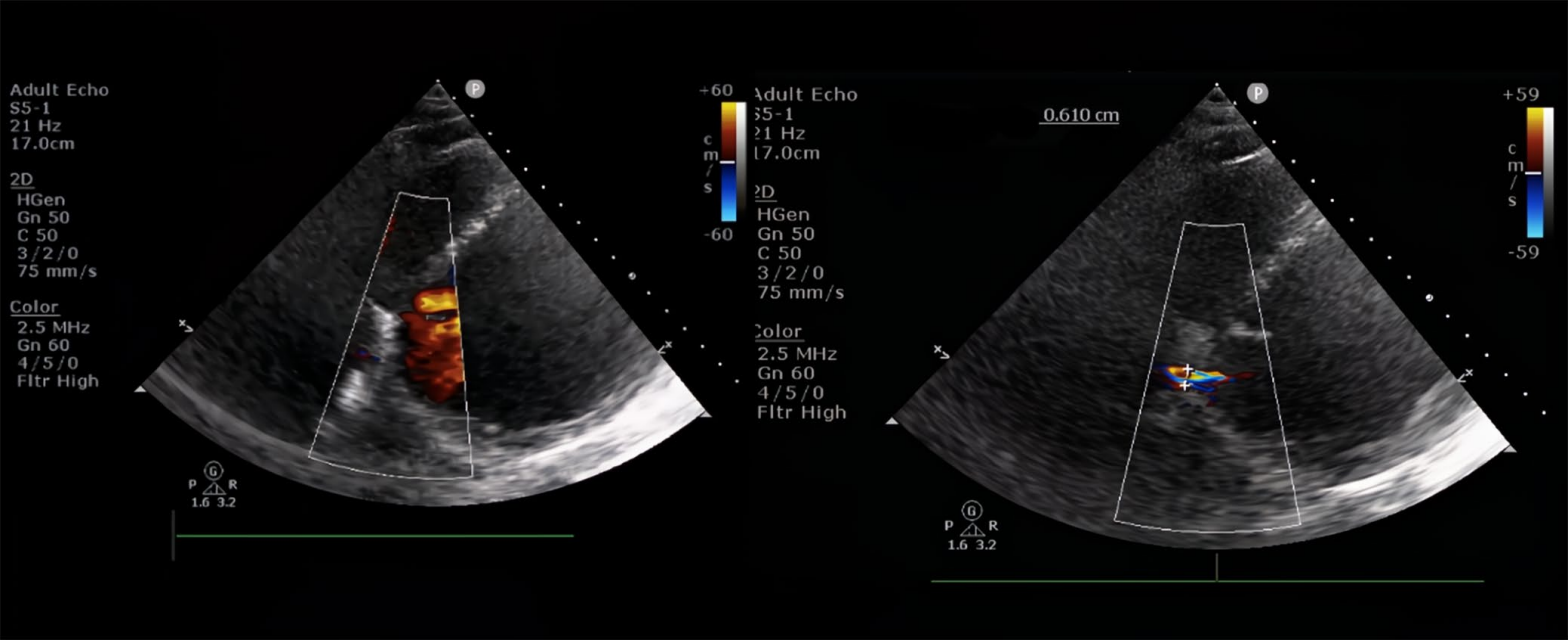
Transthoracic echocardiography 3 days after procedure showing shunt on puncture site.
